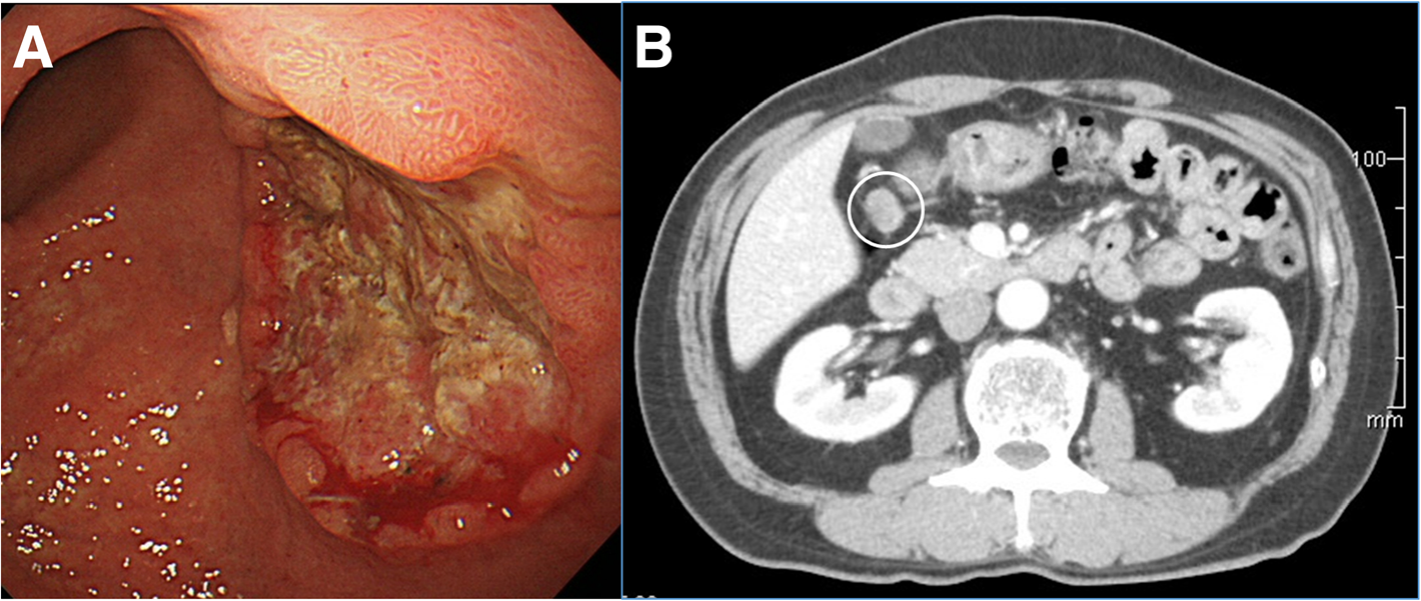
Esophagogastroduodenoscopy and computed tomography of the tumor. a Esophagogastroduodenoscopy revealed a type 2 lesion in the posterior wall of the lower body of the stomach. b Contrast-enhanced computed tomography indicated swelling of the perigastric lymph node (white circle) but showed no other distant metastasis
